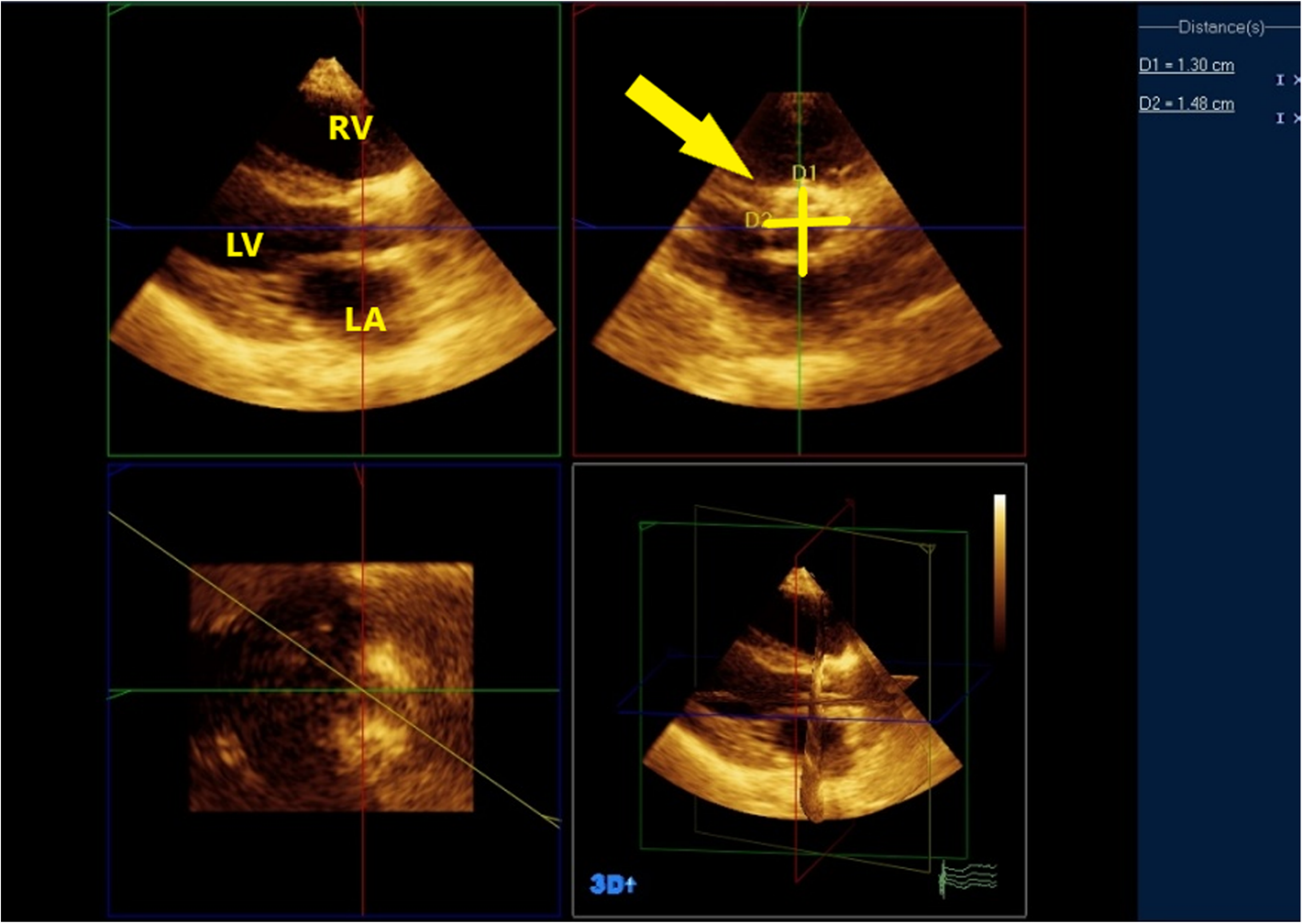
Aortic annulus was measured by 3D echocardiography in three orthogonal planes using MPR mode in patient no. 1
